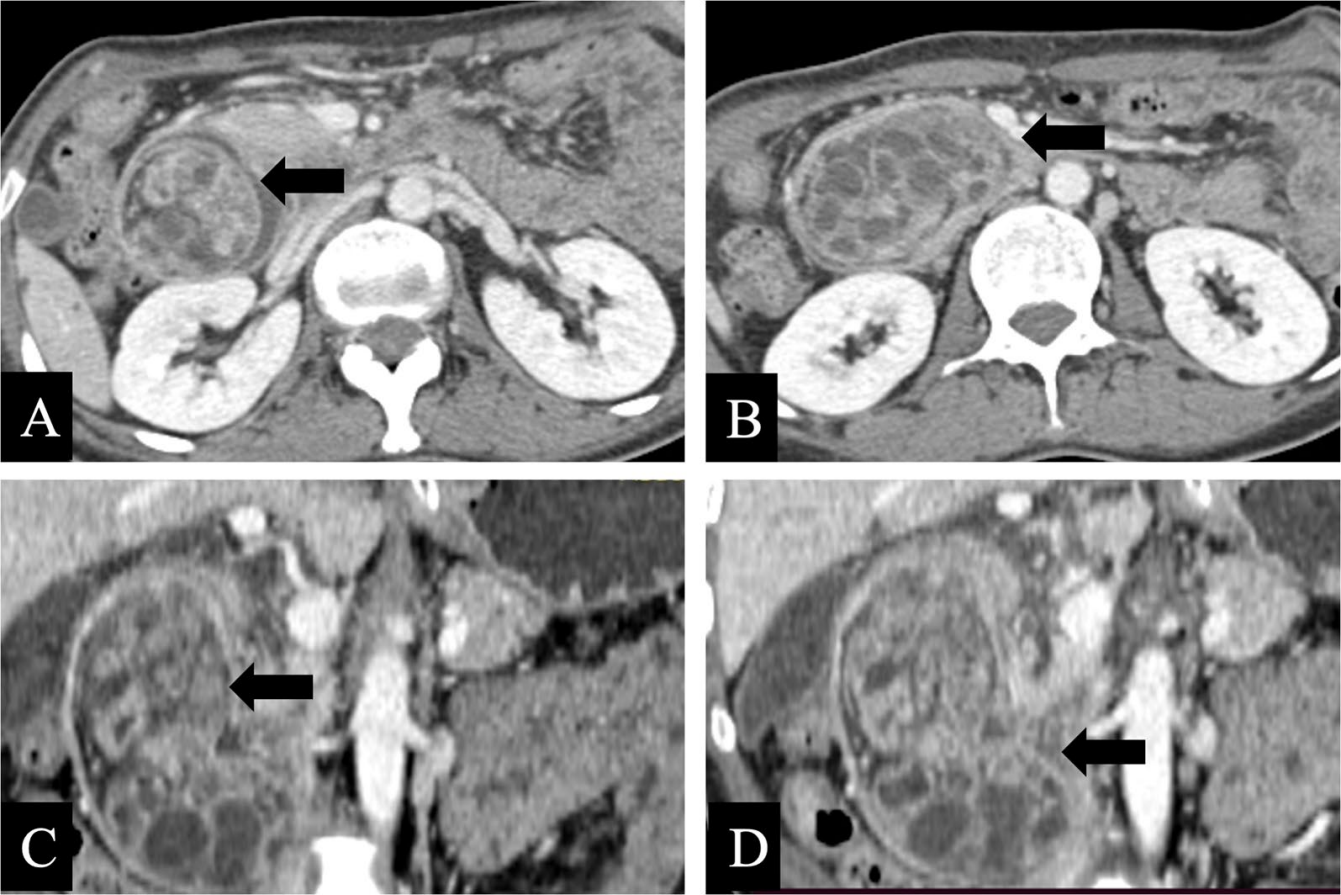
a–d A 46-year-old female presented with abdominal pain and anorexia over the course of one year with a weight loss. Contrast-enhanced axial CT images presented a 5.8 × 4.9 cm, endoluminal, and ill-defined mass (black arrows) in the duodenum accompanied by subsequent gastric outlet obstruction with surrounding inflammation and duodenal intussusception, and the patient was noted to have mild elevation in her amylase and lipase. The patient was subsequently taken to the operating room for antrectomy and Billroth II reconstruction. Her pathology demonstrated a heterotopic pancreas in the duodenum
